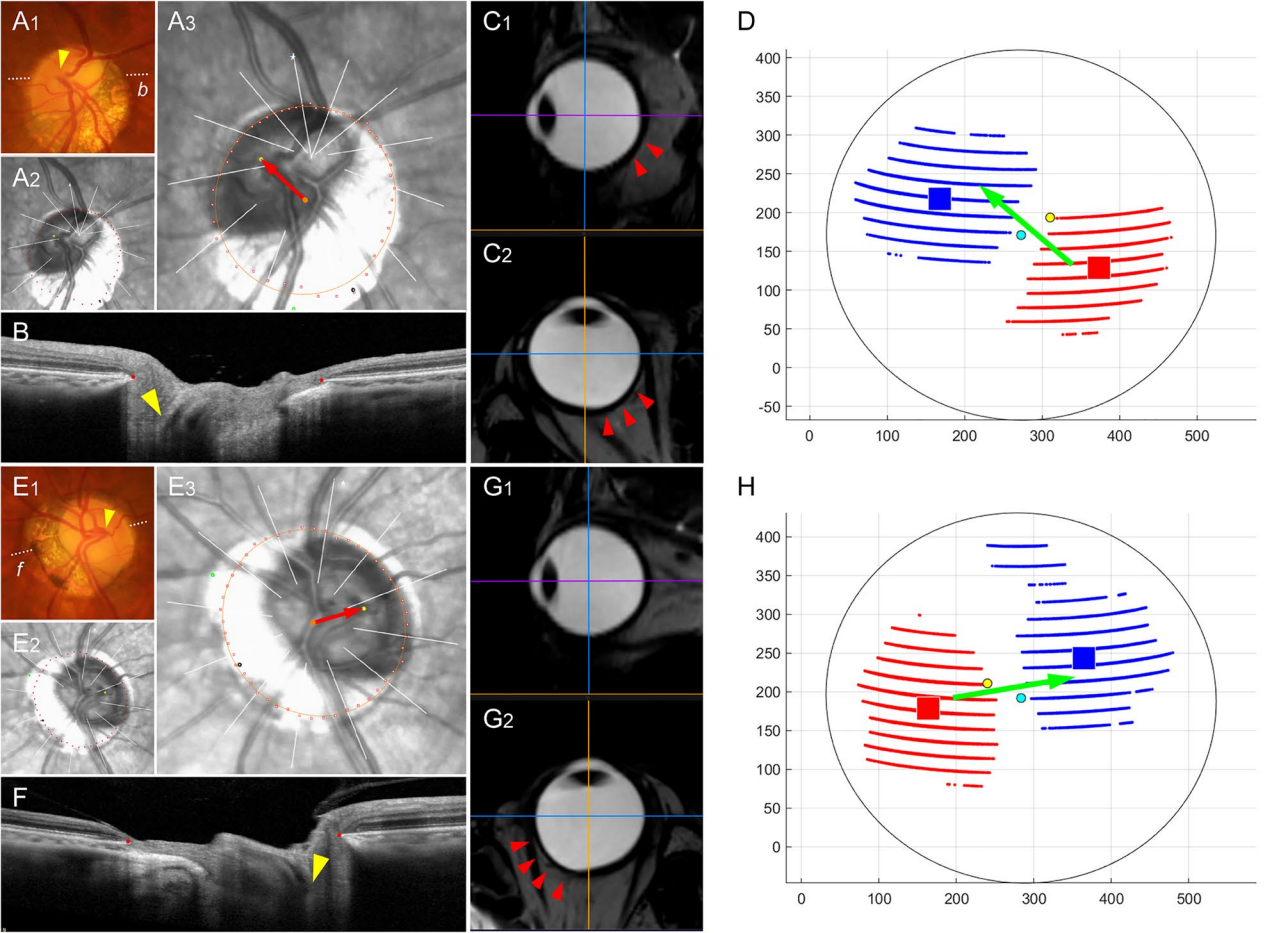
Sample cases of intra-individual difference. (A–D) Right and (E–H) left eyes of same subject. (A_1_, E_1_) Disc photographs. The arrowheads indicate the locations of the central retinal vascular trunk (CRVT). The dotted white lines indicate the locations of the OCT scans. (A_2_, E_2_) Infrared images with demarcated Bruch’s membrane opening (BMO) margin (red dots). The CRVT positions (yellow dot), the meridians of the longest externally oblique border (EOB, black dot), and the meridians of the maximal width of β-zone parapapillary atrophy (PPA, green dot) are marked on the same infrared images. (A_3_, E_3_) Measurement of lamina cribrosa (LC) / Bruch’s membrane opening (BMO) offset. The red arrows are drawn to show the direction and extent of CRVT (yellow dots) deviation from the BMO center (orange dots). (B, F) B-scan images. The red dots indicate the BMO margin and the yellow arrowheads indicate the CRVTs. (C, G) Sagittal and transverse sectional images of three-dimensional (3D) MRI. Asymmetric expansion is noted by the red arrowheads (D, H) 3D eyeball shapes are evaluated by optimal ellipsoid fitting. The blue dots indicate the location of the fovea, and the yellow dots that of the BMO. The red squares indicate the outermost protruded points, and the blue squares indicate the reciprocal innermost depressed points. Please note that the LC/BMO offset direction could differ between paired eyes of the same individual, and that it is determined by the 3D shape of the eyeball from the overgrowth to undergrowth directions (green arrows).
